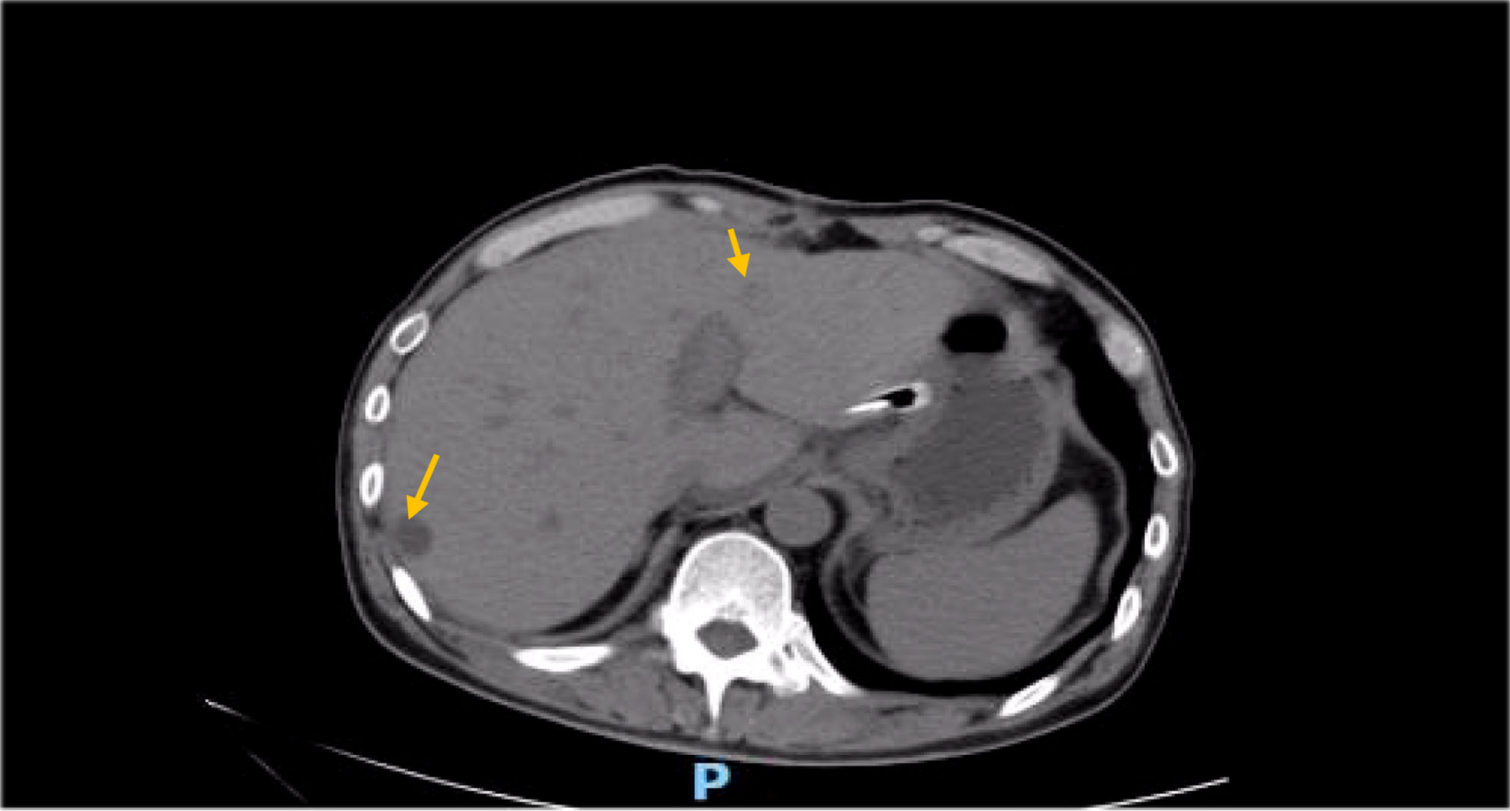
CT (liver metastasis) was performed.
